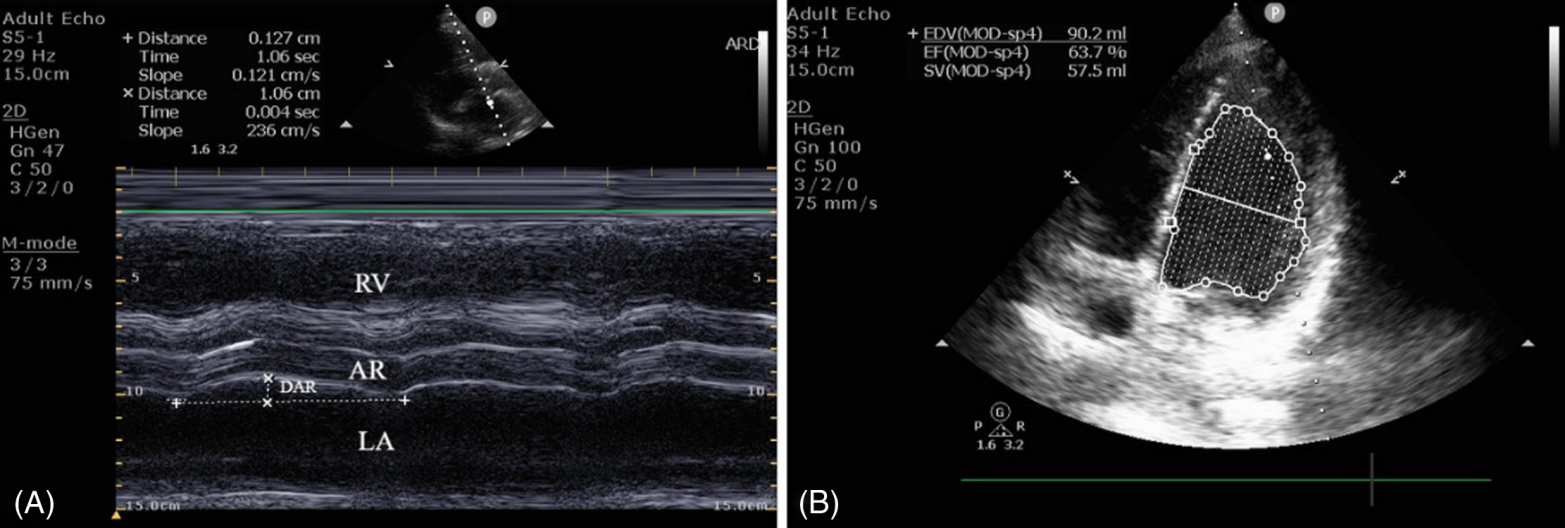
(A) We assessed ejection fraction (EF) at the bedside using M-mode ultrasonography, measuring the displacement of the aortic root (DAR) in the parasternal long-axis view. The recorded DAR for this patient was 1.06 cm. We calculated the EF using the formula (EF = 20 + 44 × 1.06), which resulted in 66.6%. (B) The echocardiographers calculated EF using the modified Simpson method [(EDV − ESV) / EDV], where [(90.2 − 32.7) / 90.2] × 100 resulted in an EF of 63.7%.
